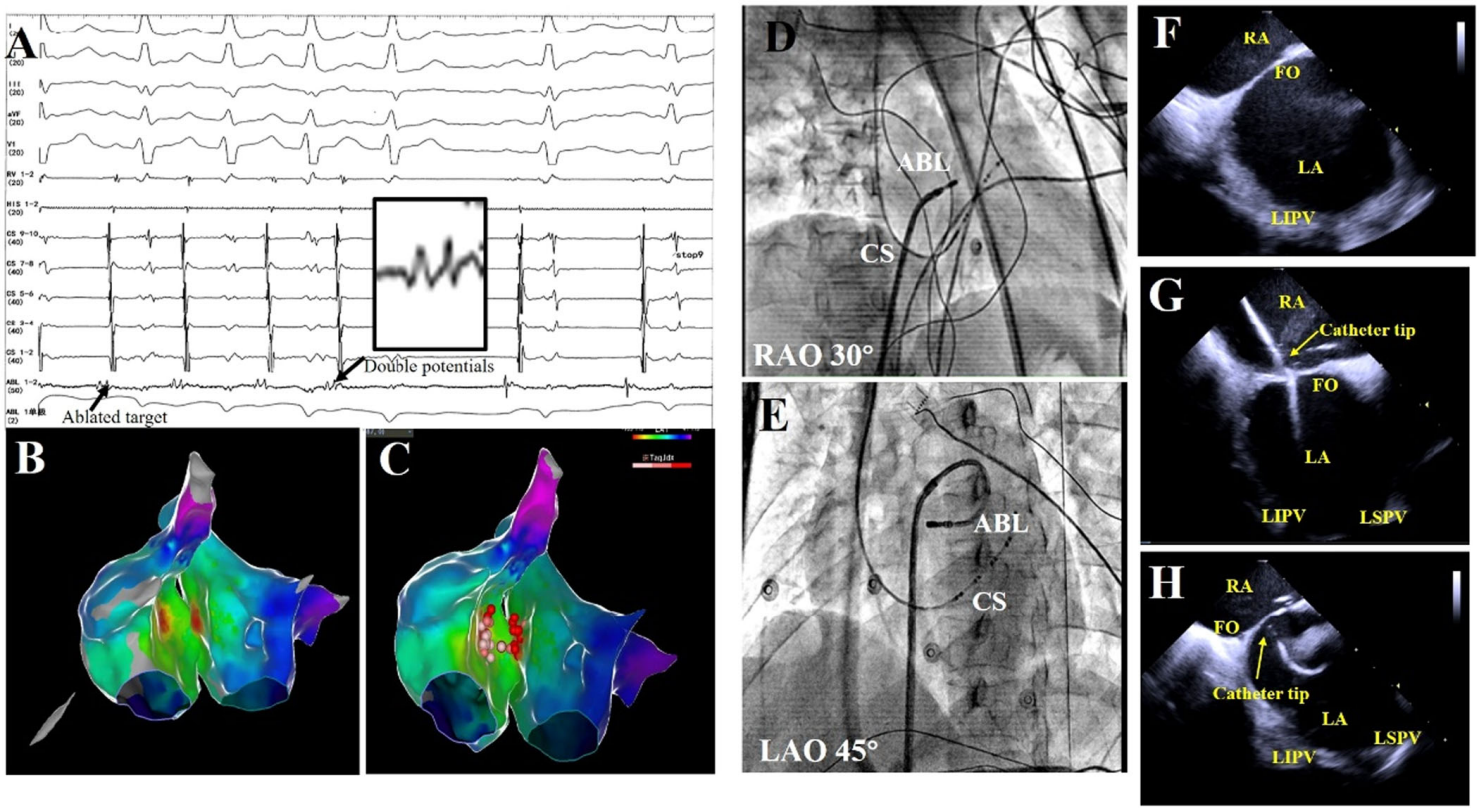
The ablation targets for a patient with FAT originating from FO in the Bilateral Ablation Group. (A) Cycle lengths of FAT in intracardiac electrogram and the ablation catheter on the right‐sided FO with double potentials. (B) Three‐dimensional electroanatomic map showing the ablated target of FAT in this group. The earliest atrial activation was recorded at the right and left atrium of FO. (C) Ablation performed at both the right and left earliest sites. (D) Fluoroscopic view of the successful right‐sided ablation site of FO at the left anterior oblique 45°. (E) Fluoroscopic view of the successful left‐sided ablation site of FO at the left anterior oblique 45°. (F) Intracardiac ultrasound image showing the FO. (G) Intracardiac ultrasound demonstrating the catheter tip contact with the right‐sided FO. (H) Intracardiac ultrasound demonstrating the catheter tip contact with the left‐sided FO.
